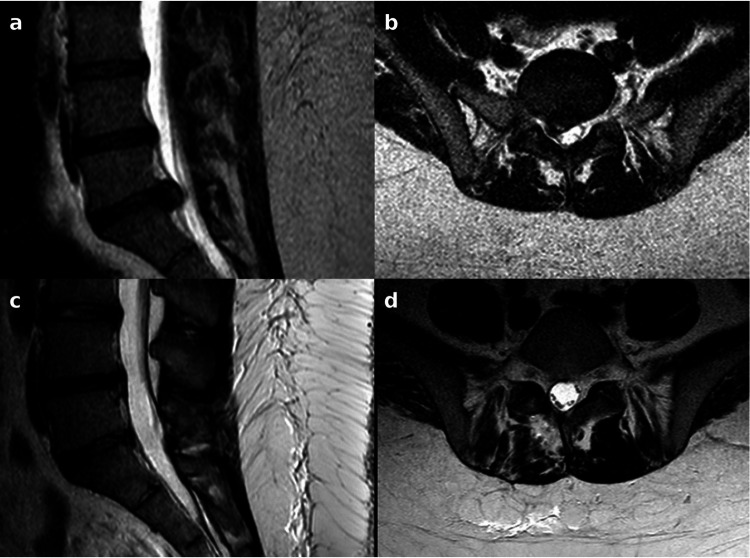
Pre- and postoperative MRI of a 16-year-old female with a right L5–S1 lumbar disc herniation treated by right L5–S1 minimally invasive microdiscectomy (MIS-MCD). (a) Preoperative T2-weighted sagittal MRI; (b) preoperative T2-weighted axial MRI; (c) postoperative T2-weighted sagittal MRI; (d) postoperative T2-weighted axial MRI
